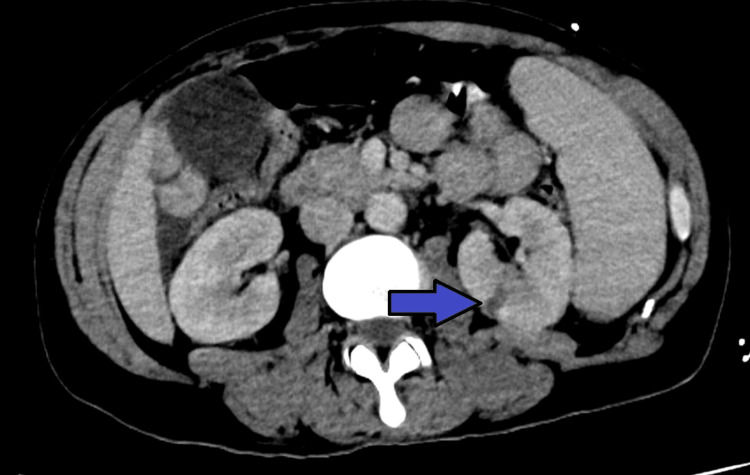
Abdominopelvic CT scan showing a left renal infarction (blue arrow).
